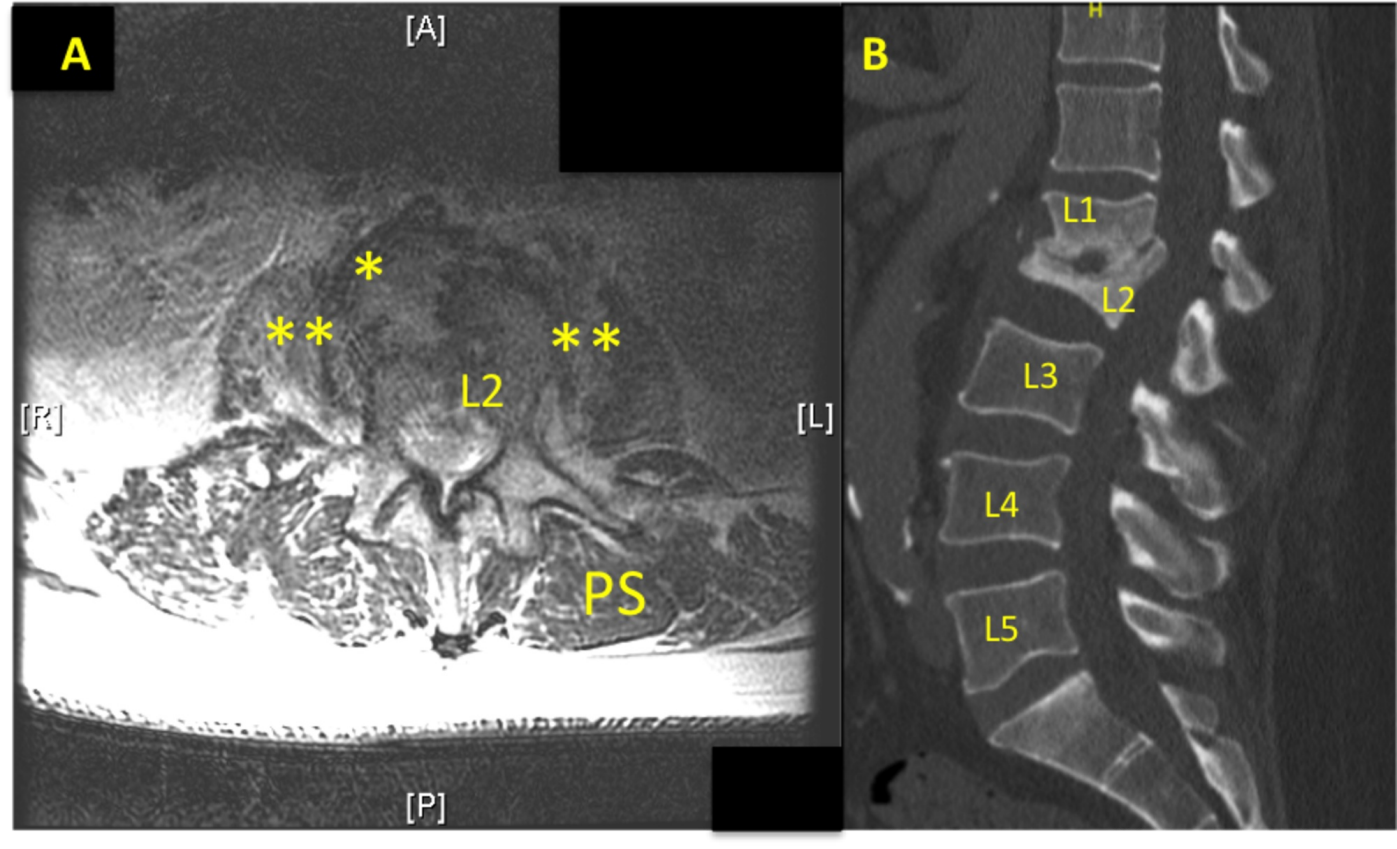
Re-admission MRI Lumbar and CT Lumbar Re-admission, Figure 2A: (MRI T2 wo contrast, axial): Non-diagnostic as the patient terminated the study after three sequences. Mild improvement in paravertebral abscess (yellow *). Psoas muscles (double yellow *). Paraspinal muscles (PS). Figure 2B (CT lumbar w contrast) Gibbus deformity at this level, discitis most likely reflecting progression of infection. Comparison to prior, progression of loss of vertebral body height of L2 and L1.
